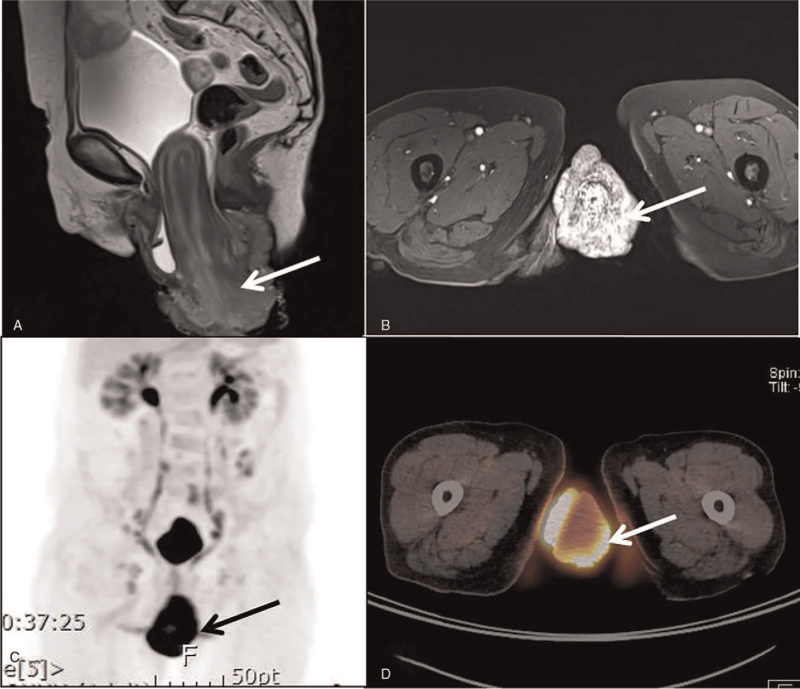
Imaging findings of squamous cell carcinoma (verrucous) of cervix and complete uterine prolapse. (A) T2-weighted sagittal magnetic resonance (MR) image showing complete prolapse of uterus and vagina (arrow). (B) Contrast-enhanced axial T1-weighted MR image showing marked enhancement in the prolapsed vagina and cervix (arrow). (C, D) FDG PET/CT images showing increased FDG uptake (arrow) in the prolapsed vagina and cervix. FDG = 18F-fluorodeoxyglucose, PET-CT = positron emission tomography-computed tomography.
